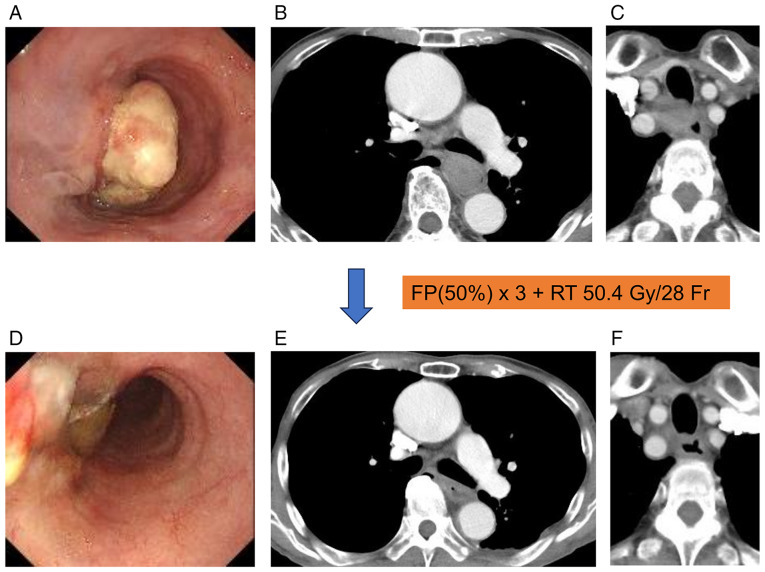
Findings of the EGD and CT scans before and after CRT. (A) EGD revealed an elevated tumor in the esophagus at a distance of 30–35 cm from the incisors. (B) Enhanced CT revealed a tumor located in the middle thoracic esophagus with invasion of the left main bronchus. (C) Enhanced CT revealed bulky lymph nodes (nos. 101R and 106recR) invading the trachea. (D) After CRT, EGD revealed shrinkage of the primary esophageal tumor. (E) Post-CRT enhanced CT demonstrated reduction of the primary esophageal tumor. (F) Post-CRT enhanced CT demonstrated shrinkage of the metastatic lymph nodes. FP, 5-fluorouracil + cisplatin; RT, radiation; Fr, fraction; EGD, esophagogastroduodenoscopy; CT, computed tomography; CRT, chemoradiotherapy.
